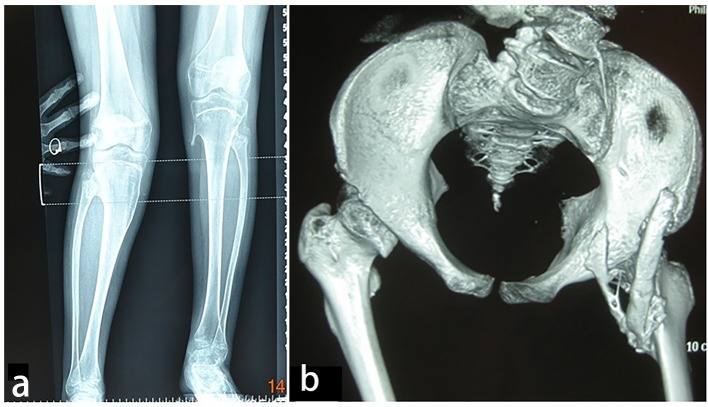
(a) Progressive episodes of heterotopic ossifications typically lead to ankylosis of all major joints of the axial and appendicular skeleton, rendering movement impossible. AP radiograph of the knees showed a 13-year-old girl presented with progressive painful limitation of range of motion of the both knees with progressive extra-severe articular ankylosis of the left ankle joint. (b) 3D reconstruction CT scan of the pelvis of the same patient (13-year-old) showed two ossified bands originated from the posterior aspect of the right iliac bone, run downwards and where both got fused and inserted beneath the femoral neck adding extra dilemma to the patient.
